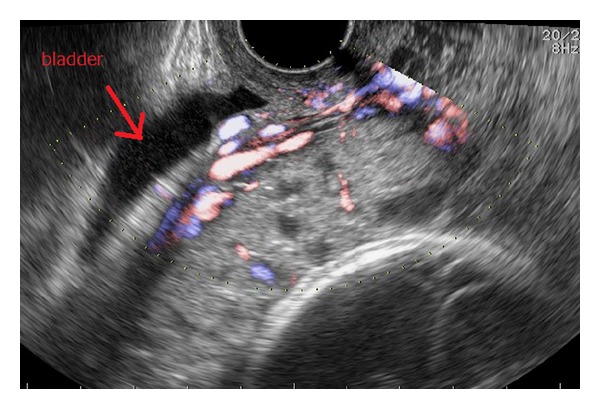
At 24 weeks of gestation. Transvaginal ultrasonography revealed loss of hypoechoic appearance of the retroplacental zone, lacunas in the placenta, and bulging of the bladder.
